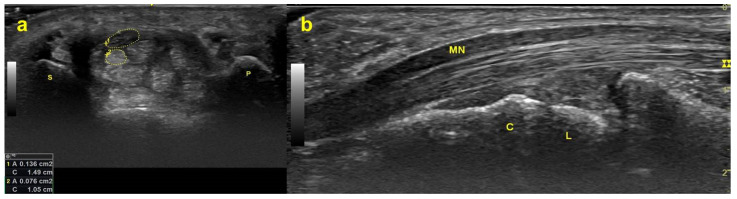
Ultrasonograms captured at the distal wrist crease level. (a) MN (structure enclosed by the upper dotted line) and FDS tendon (structure enclosed by the lower dotted line) in the carpal tunnel on the transverse plane. (b) MN and FDS tendon on the longitudinal plane. Abbreviations: P = pisiform bone; S = scaphoid bone; MN = median nerve; FDS = flexor digitorum superficialis; L = lunate bone; C = capitate bone.
